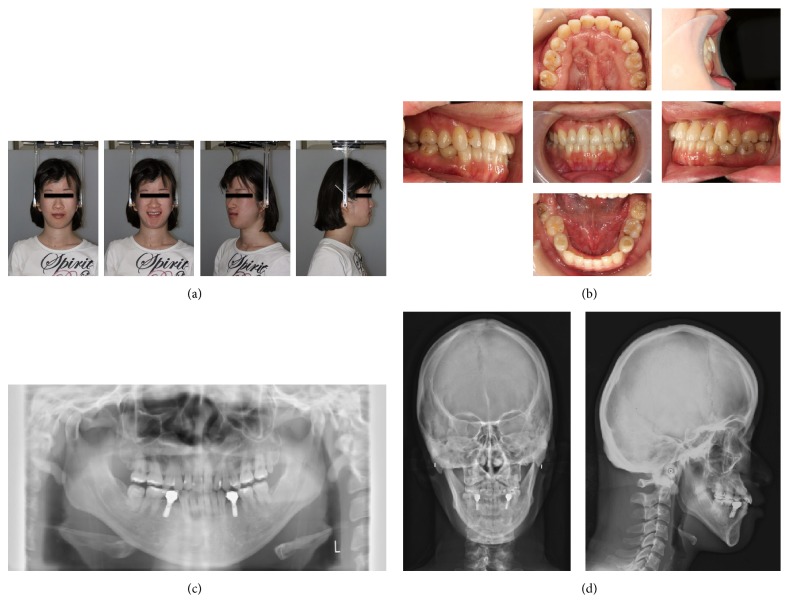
Extraoral and intraoral photographs at 2 years and 8 months after debonding (24 years and 9 months). (a) Extraoral photographs. (b) Intraoral photographs. (c) Orthopantomograms. (d) Posteroanterior and lateral cephalograms.
